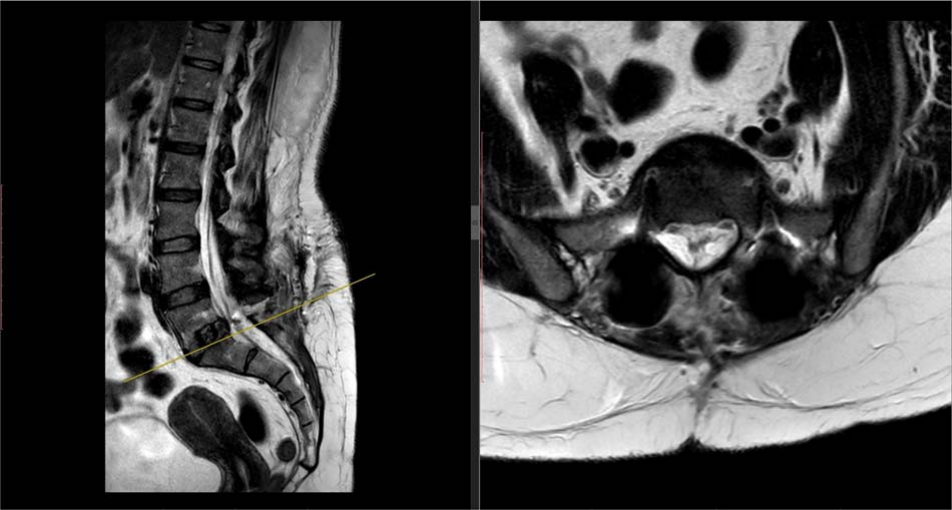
Postoperative MRI of the lumbar spine performed in January 2017. Interference from the metallic pedicular screws limited the scans clarity.
